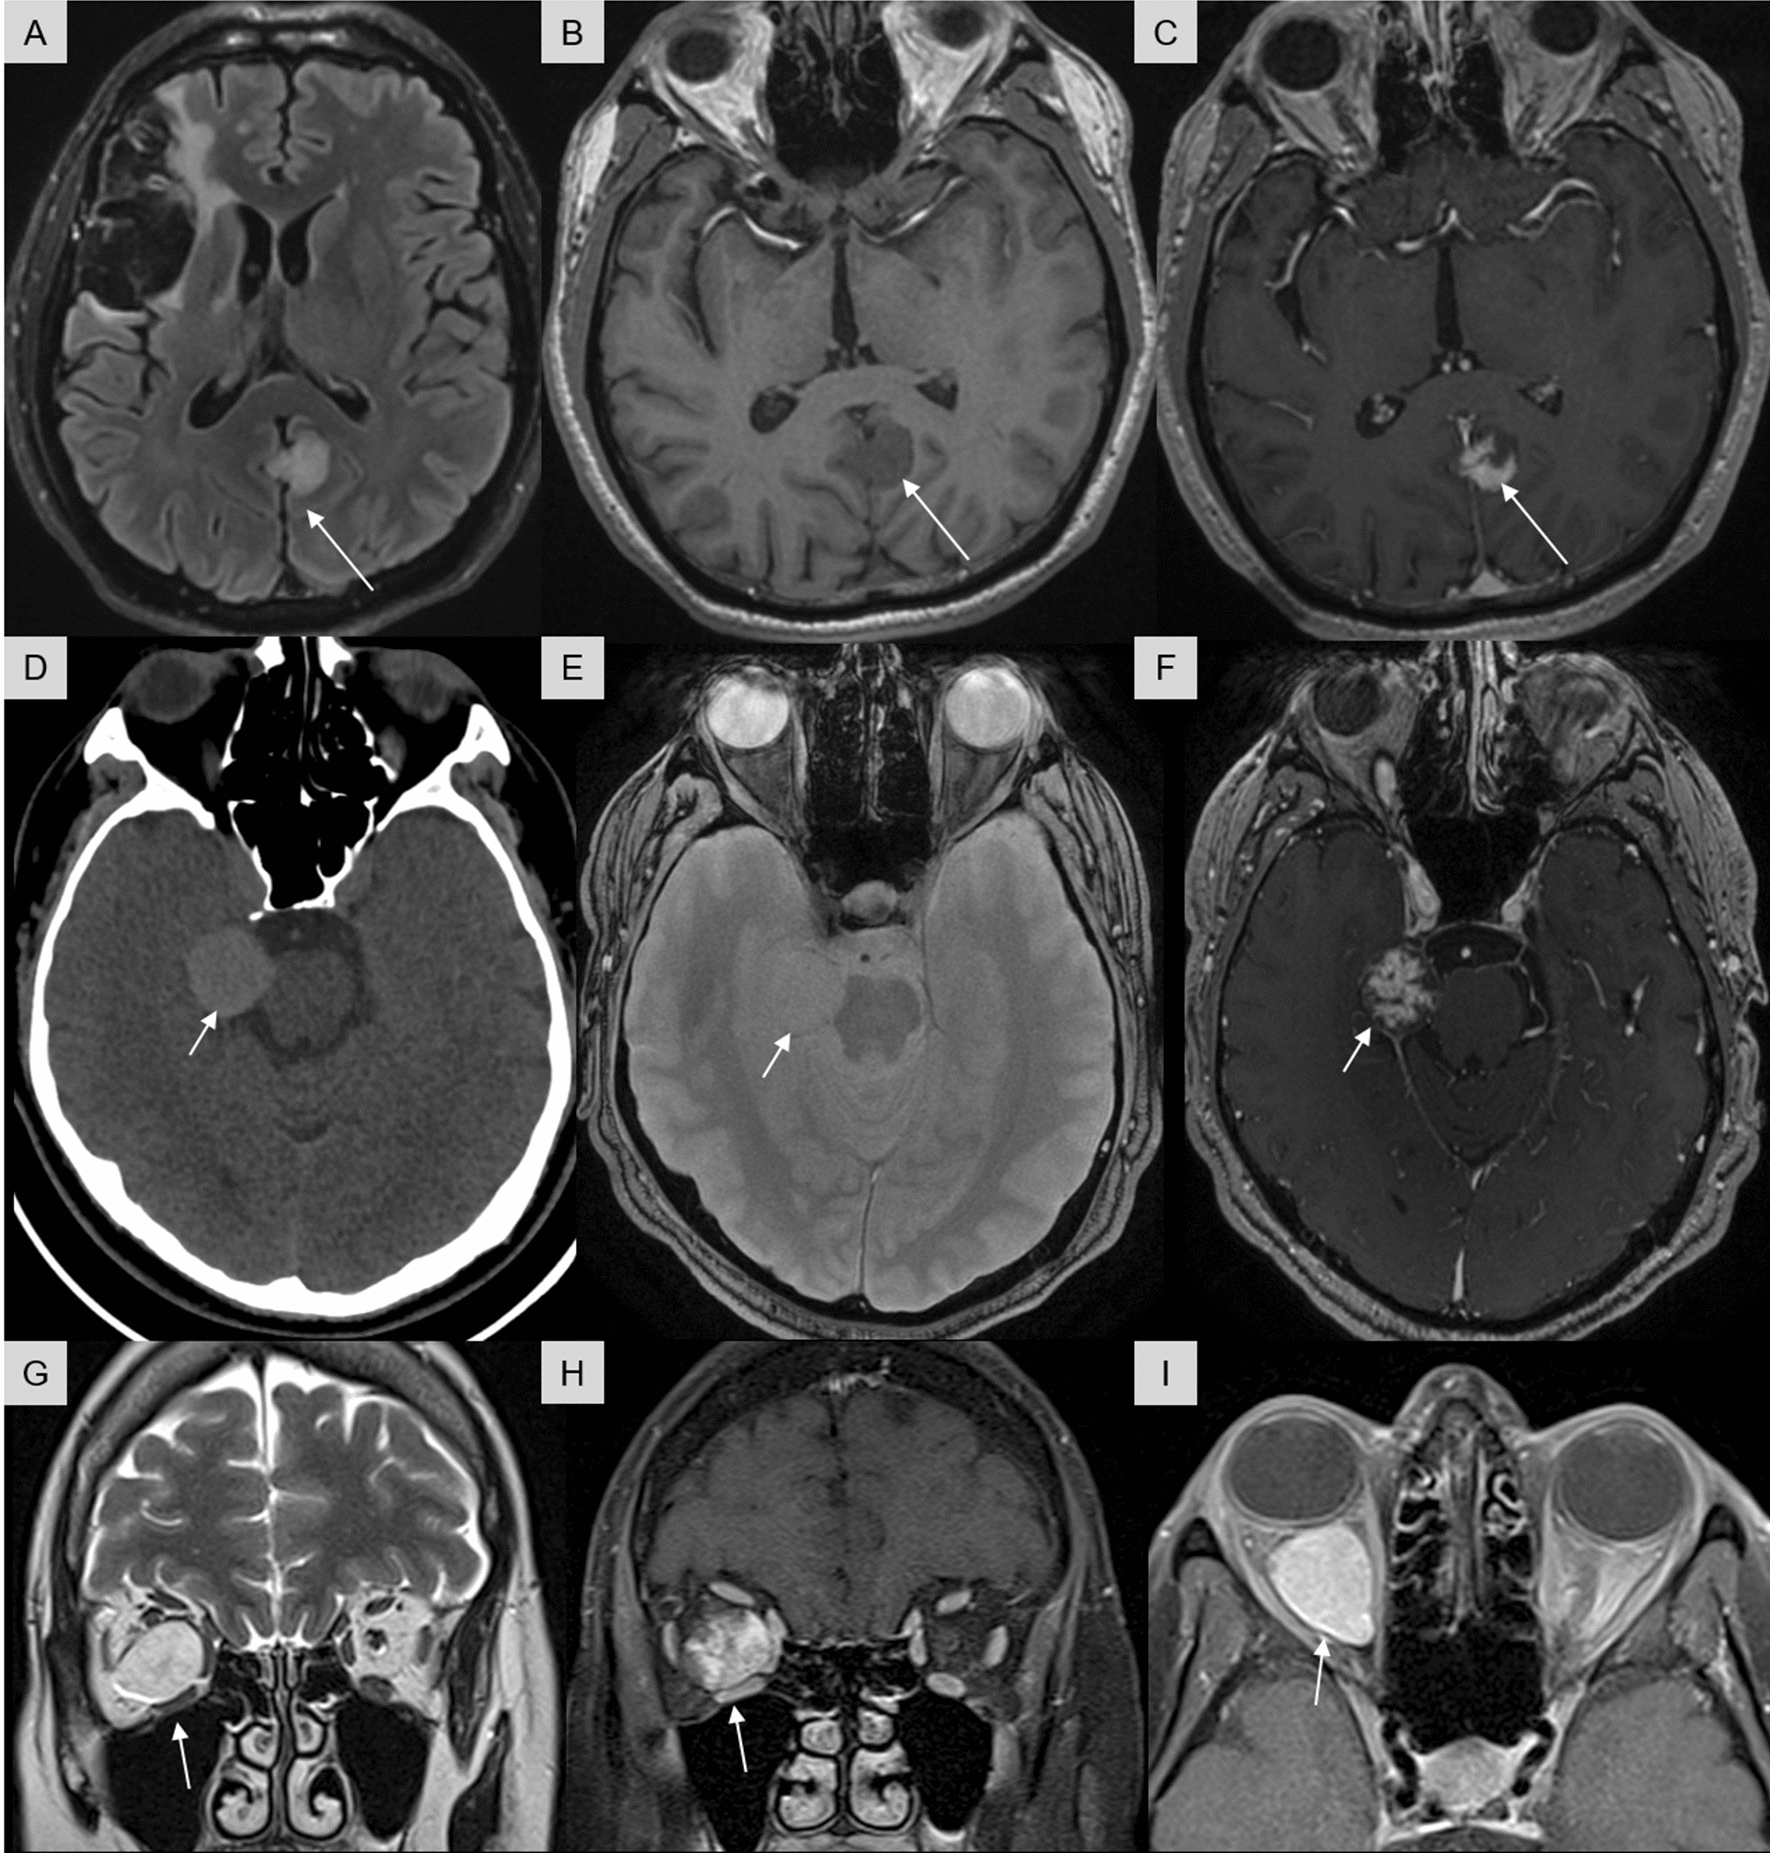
Radiological findings of the three cases of our cohort. Each line represents one patient (cases #1 to #3 from top to bottom). (A) Axial Flair sequence showing a homogeneous hyperintense extra-axial mass, well-circumscribed, in the left parietal hemisphere at the level of the posterior third of the falx, without a surrounding parenchymal edema, and the lesion of the right superficial middle cerebral artery territory infarction. (B) Axial T1-weighted MR image demonstrating a slightly homogeneous hypointense extra-axial mass. (C) After administration of gadolinium, MR axial T1FS image shows a marked and heterogeneous enhancement. Absence of dural tail sign. (D) Axial computed tomographic scan showing a round, well-circumscribed, hyperdense extra-axial lesion in the right temporal hemisphere at the level of the anterior third of the cerebellar tentorium. No adjacent edema or significant mass effect. (E) Axial T2* sequence showing no haemorrhage or hemosiderin deposits. (F) After administration of gadolinium, MR axial T1FS image shows a marked and heterogeneous enhancement. Absence of dural tail sign. (G) MRI coronal T2 sequence showing a right intraorbital intraconal mass, well-circumscribed, encapsulated, hyperintense in T2 compared to muscles, lying close to the medial and inferior rectus muscles, abuting the globe, pushing the optic nerve and in contact with the dura mater of the optic nerve. (H) After administration of gadolinium, MR coronal T1FS sequence shows a slow gradual and irregular enhancement. (I) On delayed contrast enhanced-image, we can notice a full filling of the mass
